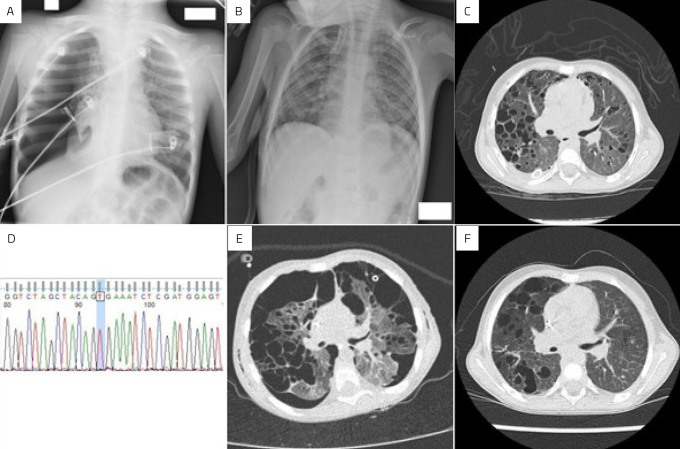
(A) X-ray of admission showed a right pneumothorax. (B) X-ray after thoracostomy, showing pulmonary expansion and diffuse interstitial lung disease. (C) CT after thoracostomy, showing diffuse cystic lung disease. (D) Electropherogram showing the absence of mutation (blue column) at codon 600 of the BRAF gene (wild-type). (E) CT at the beginning of remission therapy, during new bilateral pneumothorax. (F) CT at the end of maintenance therapy shows good pulmonary expansion and reduced number and size of pulmonary cysts.
